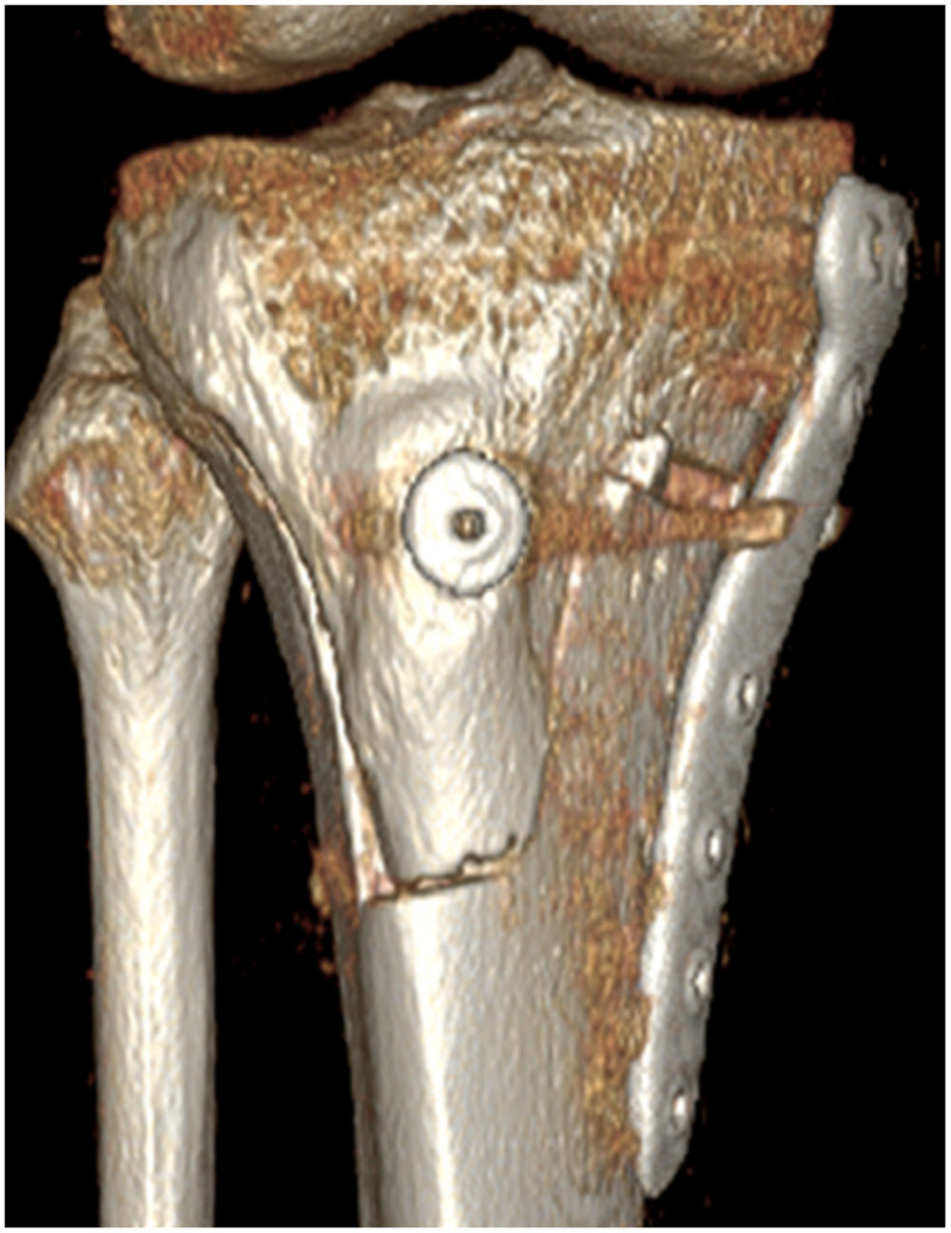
Postoperative computed tomography of open wedge distal tuberosity tibial osteotomy.
